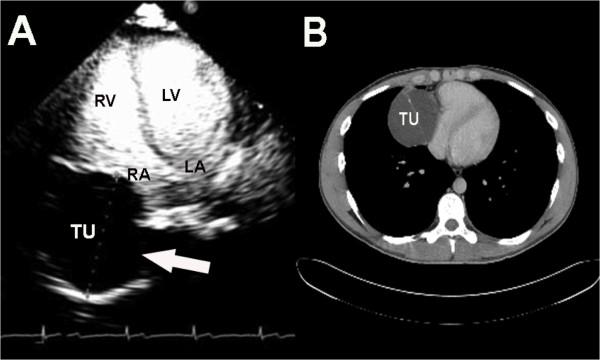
Contrast echocardiographic and computed tomographic images. (A) Contrast-enhanced echocardiographic image obtained after intravenous injection of lipid-encapsulated microbubbles. The apical four-chamber view demonstrates normal left ventricular opacification and low opacification of the tumoral mass. LA, left atrium; LV, left ventricle; RA, right atrium; TU, tumoral mass. (B) Computed tomographic image demonstrating a septate cystic mass containing septal calcification, which was continuous to the pericardium and caused extrinsic compression of the right atrium.
